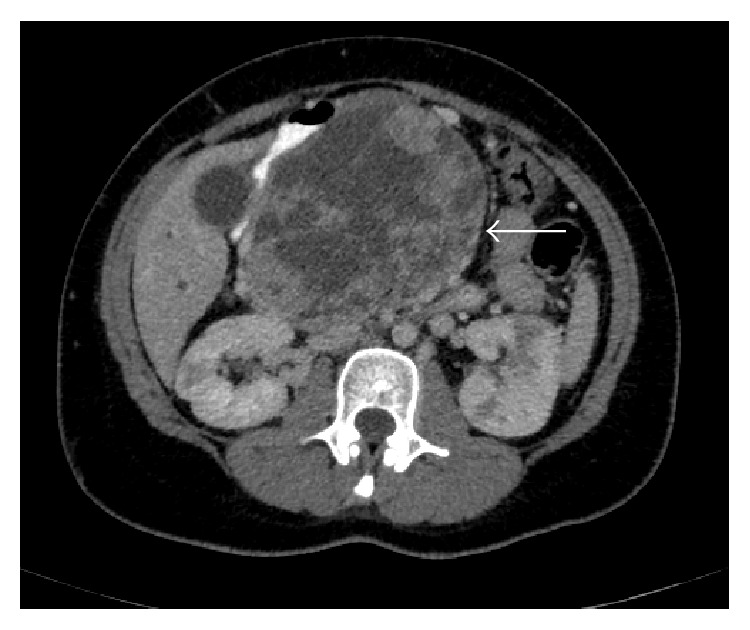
Abdominal CT reveals a voluminous lesion in pancreatic head and body (arrow).
